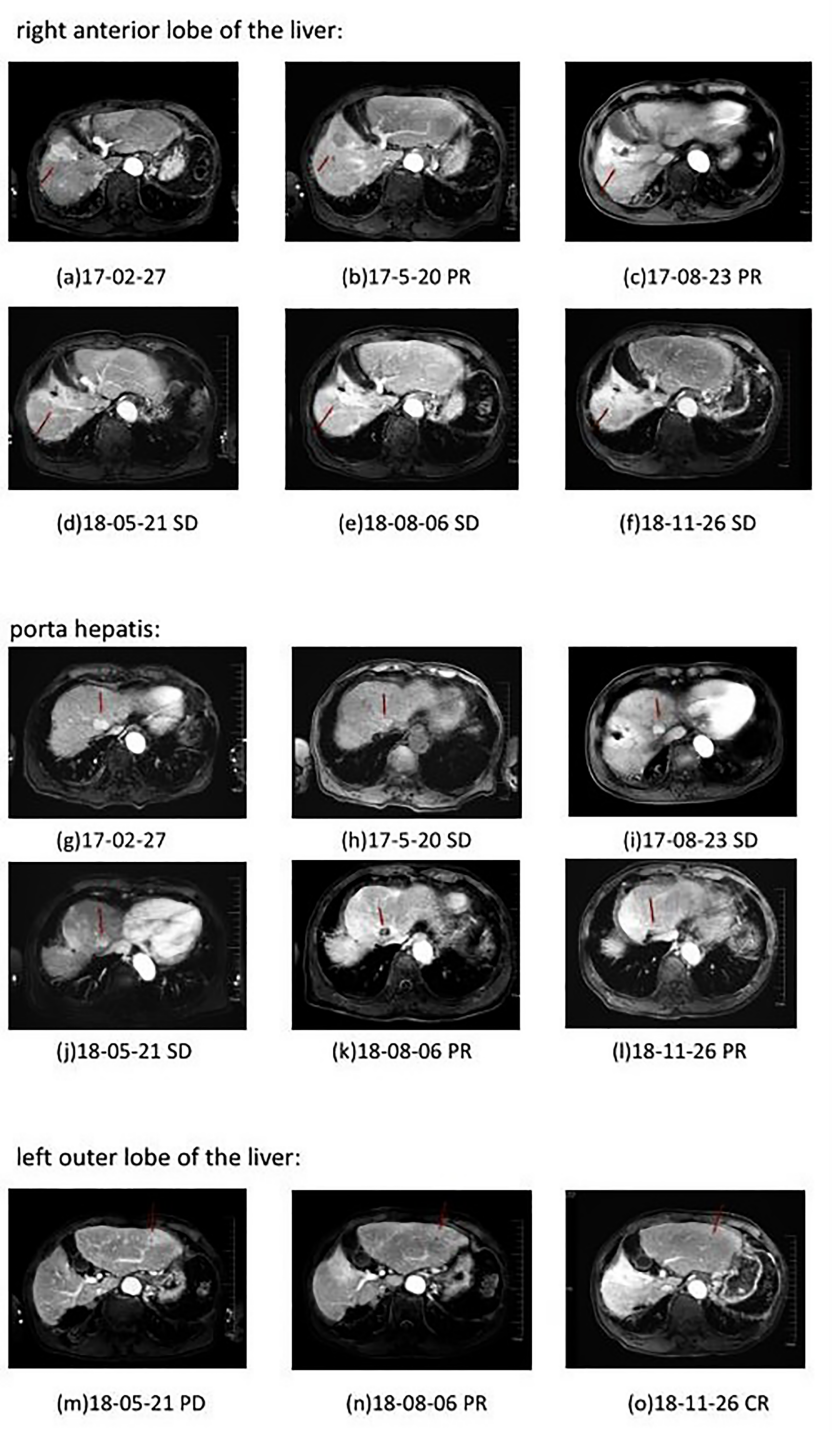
Plain and enhanced MRI of lesions in different parts of patient in different periods. a–f: right anterior lobe of the liver; g–l: porta hepatis; m–o: left outer lobe of the liver. Efficacy evaluation: PD, Disease progression; SD, Stable disease; PR, Partial response; CR, Complete response.
